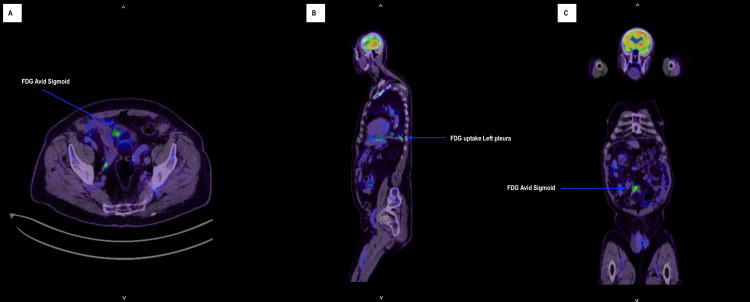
Preoperative FDG PET-CT demonstrating a metabolically active sigmoid lesion and pleural uptake (A) Axial fused FDG PET-CT image demonstrating focal increased FDG uptake within the sigmoid colon corresponding to the clinically identified lesion (arrow). (B) Sagittal fused FDG PET-CT image demonstrating increased metabolic uptake within the left pleura corresponding to areas of pleural thickening (arrow), raising suspicion for metastatic disease. (C) Coronal fused FDG PET-CT image demonstrating focal FDG uptake within the sigmoid colon consistent with the metabolically active sigmoid lesion (arrow). FDG, fluorodeoxyglucose
